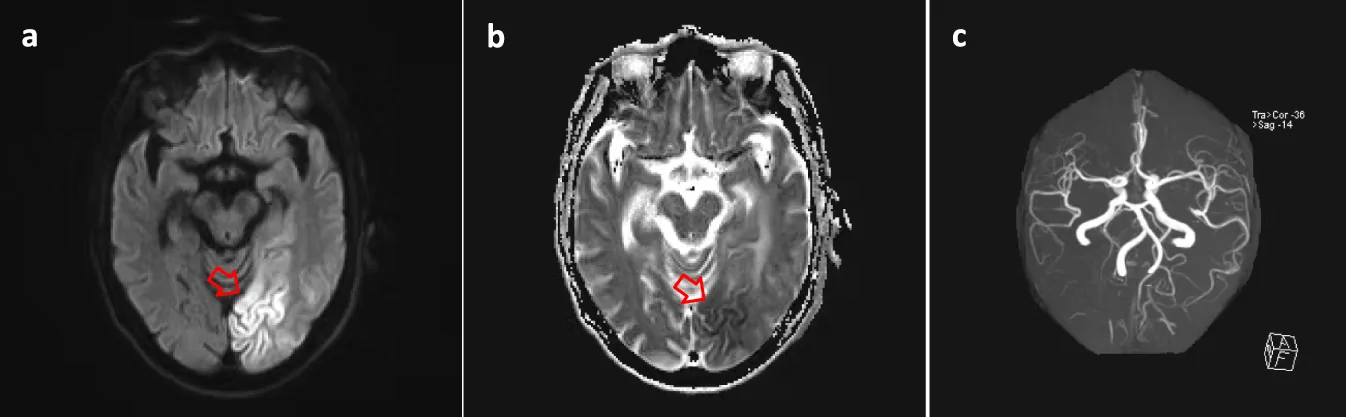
13 November 2022 MRI: (a,b) Lacey like DWI high signal and ADC low signal are observed in the left temporal occipital parietal lobe; (c) MRA did not show cerebral artery stenosis in the lesion area, which does not conform to vascular distribution.
